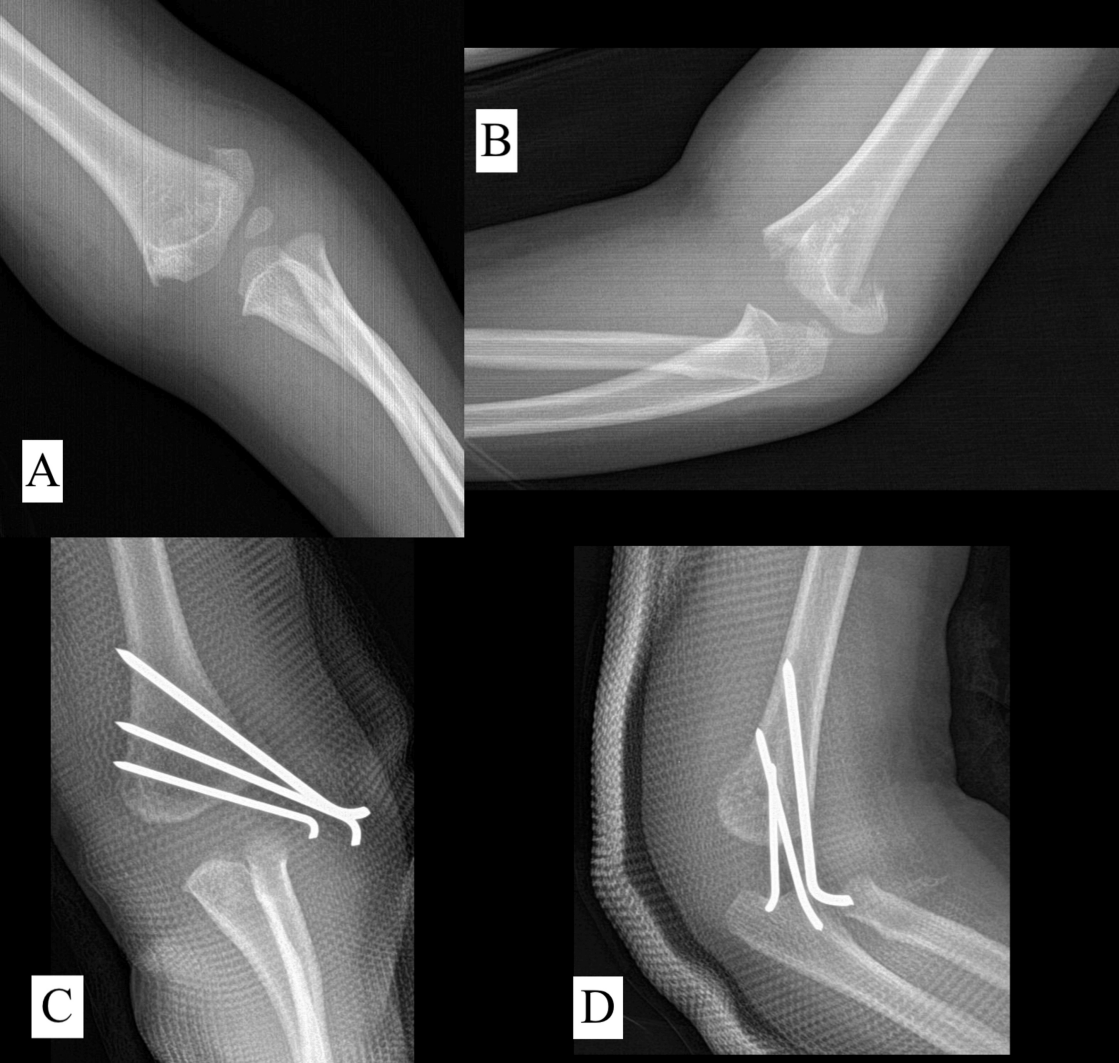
Radiograph of a three-year-old patient with a Type III supracondylar fracture (A) Preoperative AP view. (B) Preoperative lateral view. (C) Postoperative AP view showing all-lateral pin configuration. (D) Postoperative lateral view.
